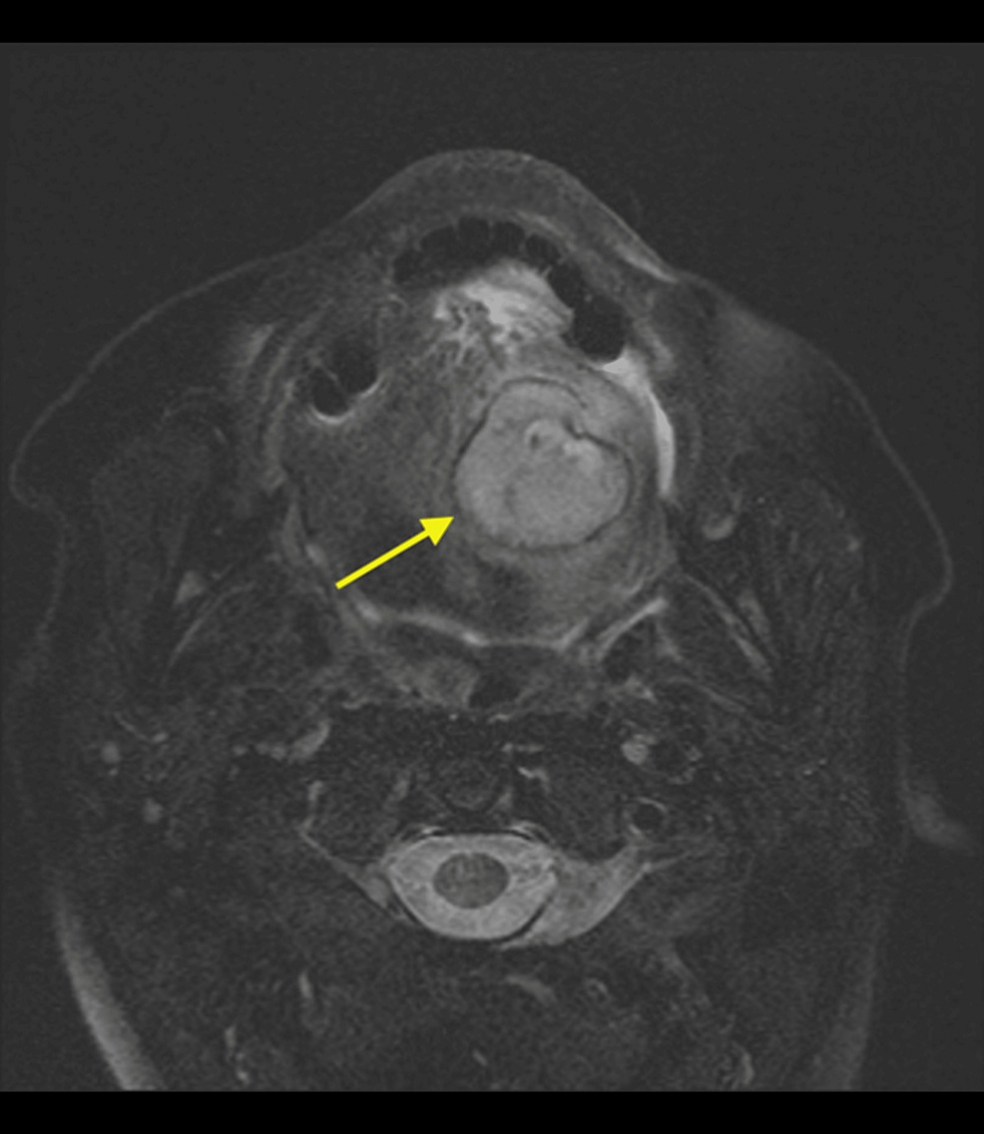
Axial fat-suppressed T2-weighted magnetic resonance image of the neck showing T2 hyperintense lesion with a thin T2 hypointense rim (arrow).
